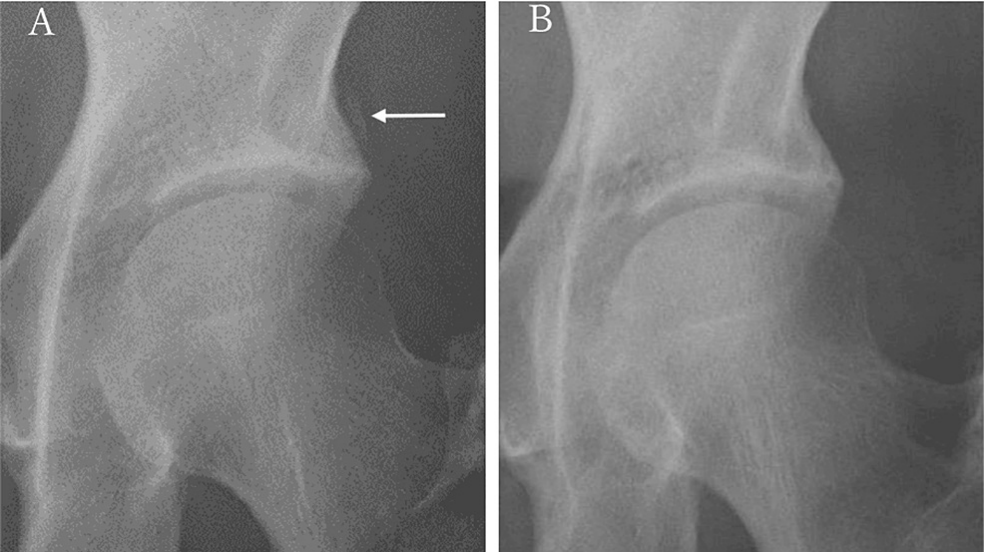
Radiograph of the left hip. Anteroposterior radiograph of the left hip showing calcification adjacent to the anterior inferior iliac spine (arrow A). Anteroposterior radiograph of the left hip after 1 month of administration demonstrated disappearance of the calcification (arrow B).
